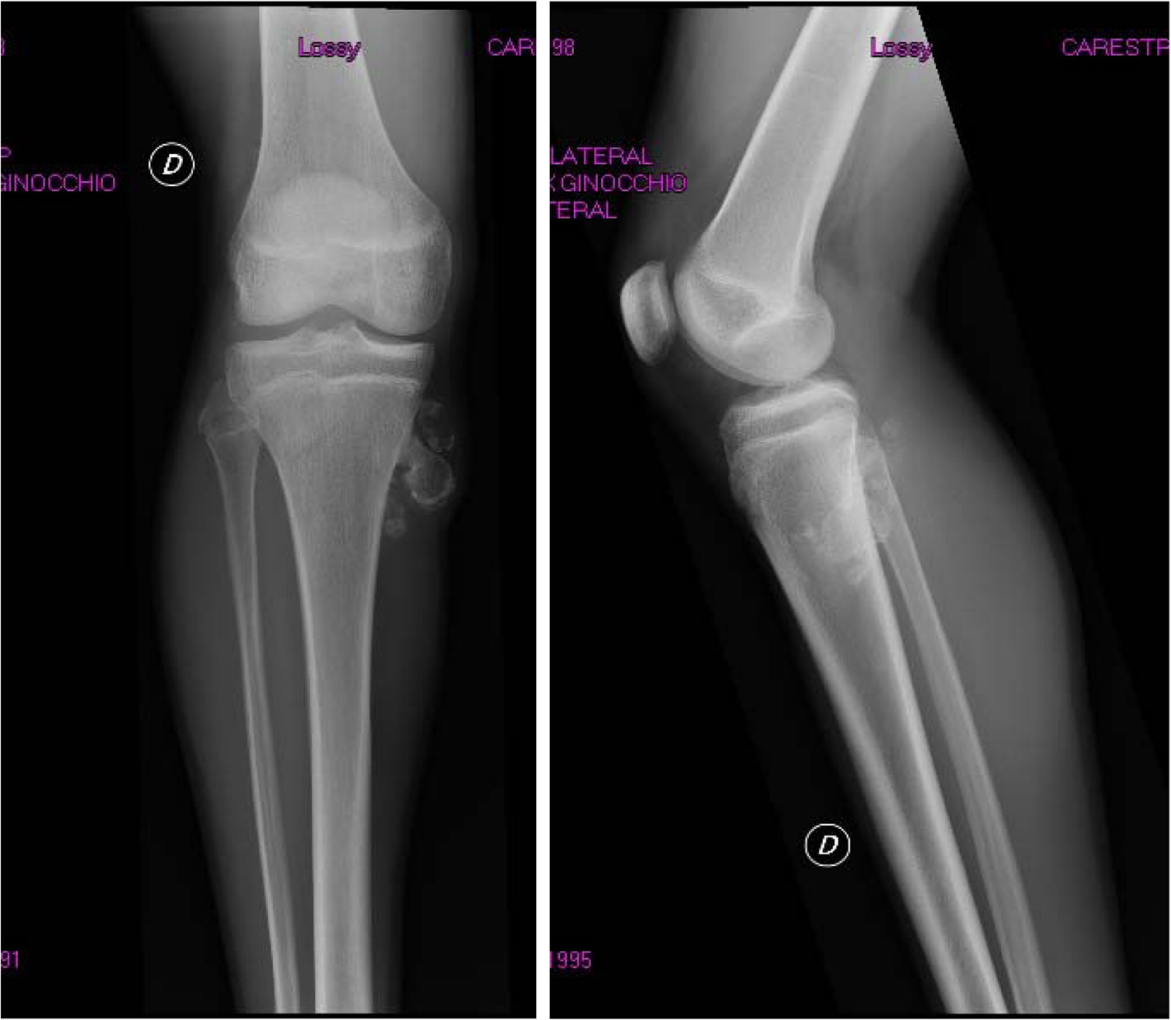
Patient 1 right leg X-rays after GH treatment
